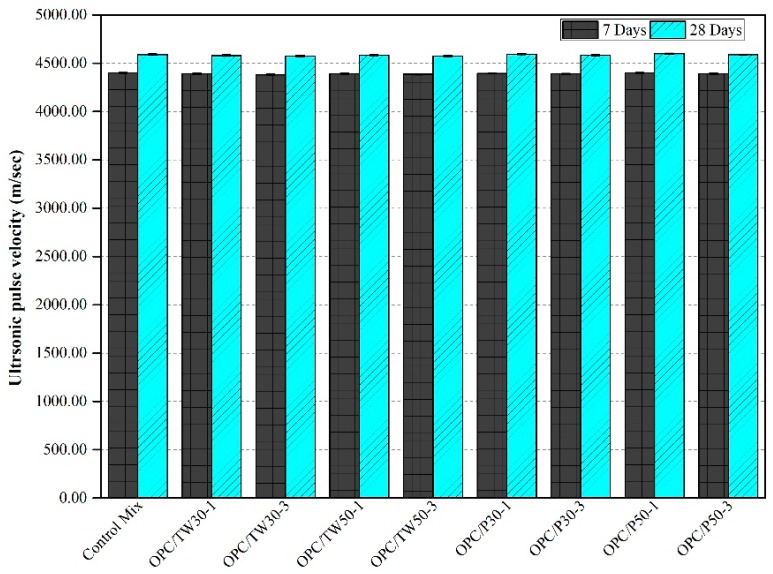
Ultrasonic pulse velocity of mortars made with tea waste and perlite particles with 100% OPC.
